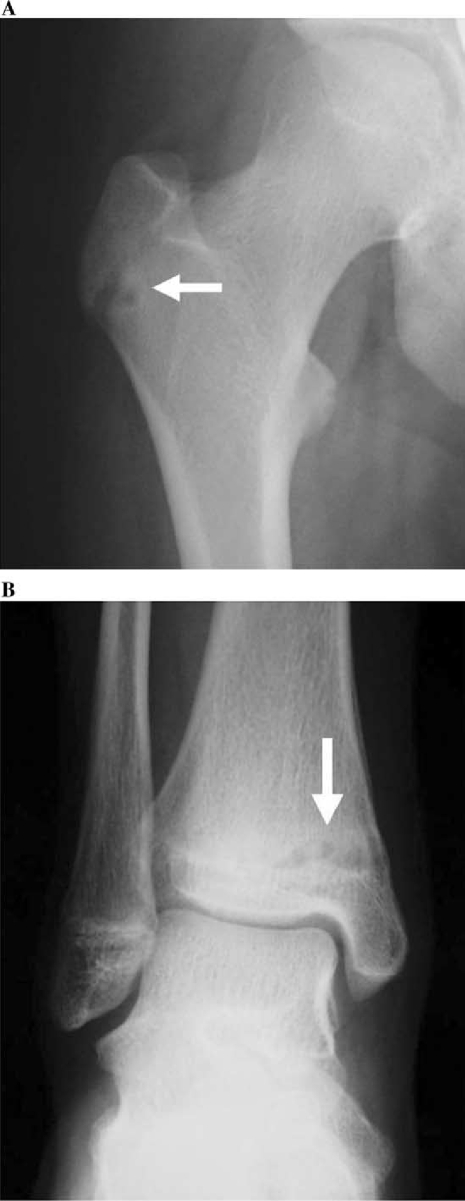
Radiographs show small and round osteolytic lesions (arrows) in the greater trochanter of the right femur (A) and the distal tibia (B).
